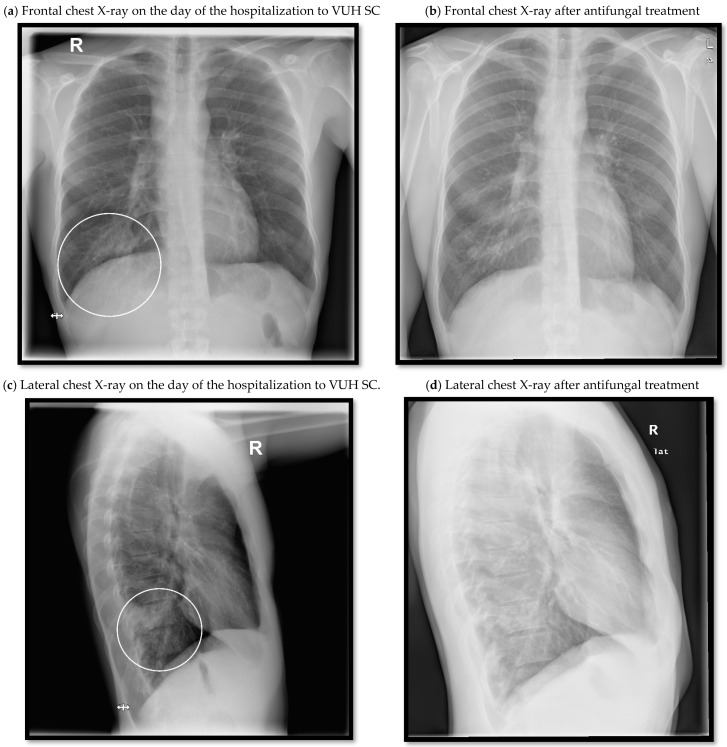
Frontal and lateral chest X-rays showing dynamics of the infiltration in the basal segment of the right lung.
